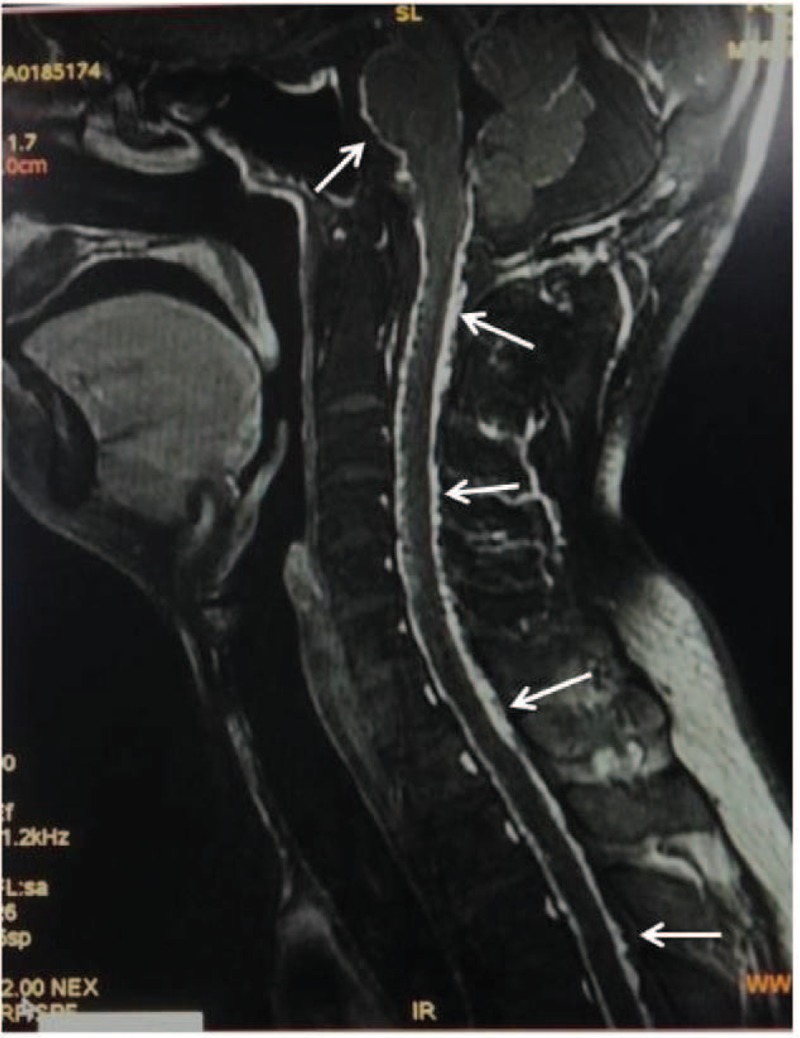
Radiological evaluation of the spinal cord. MRI T1 Gad sagittal image demonstrated the diffuse leptomeningeal enhancement (arrows). MRI = magnetic resonance imaging.
